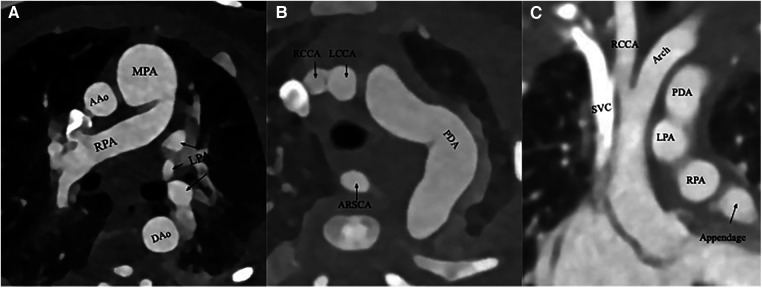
(A) An axial CTA slice depicting the right pulmonary artery (RPA) crossing the left pulmonary artery (LPA), which had already branched from the main pulmonary artery (MPA). The ascending (AAo) and descending (DAo) aortic segments are also seen. (B) An axial CTA slice demonstrating a substantial and tortuous patent ductus arteriosus (PDA) as well as an aberrant right subclavian artery (ARSCA), originating from the PDA. The other branch vessels such as the right common carotoid arteries (RCCA) and left common carotoid arteries (LCCA) are also seen. (C) A coronal CTA slice demonstrating hypoplasia of the ascending aorta and its arch. Several other structures are also seen: the PDA, RCCA, left pulmonary artery (LPA), and right pulmonary artery (RPA), and the superior vena cava (SVC).
